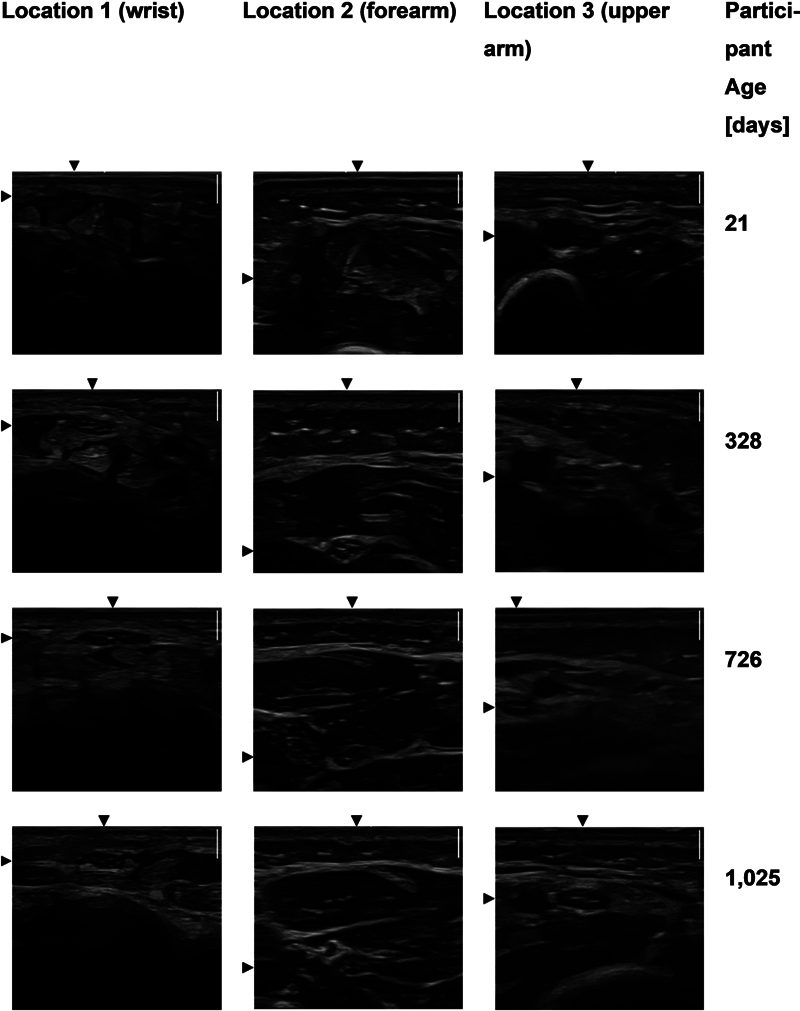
Examples of ultrasound images. This figure shows ultrasound images for locations 1, 2, and 3 for four participants of different ages (21–1,025 days). The white scale bar on the upper right side of each picture corresponds to 2 mm and applies to all of the following images. In each picture, the nerve is located at the intersection of the two arrowheads.
